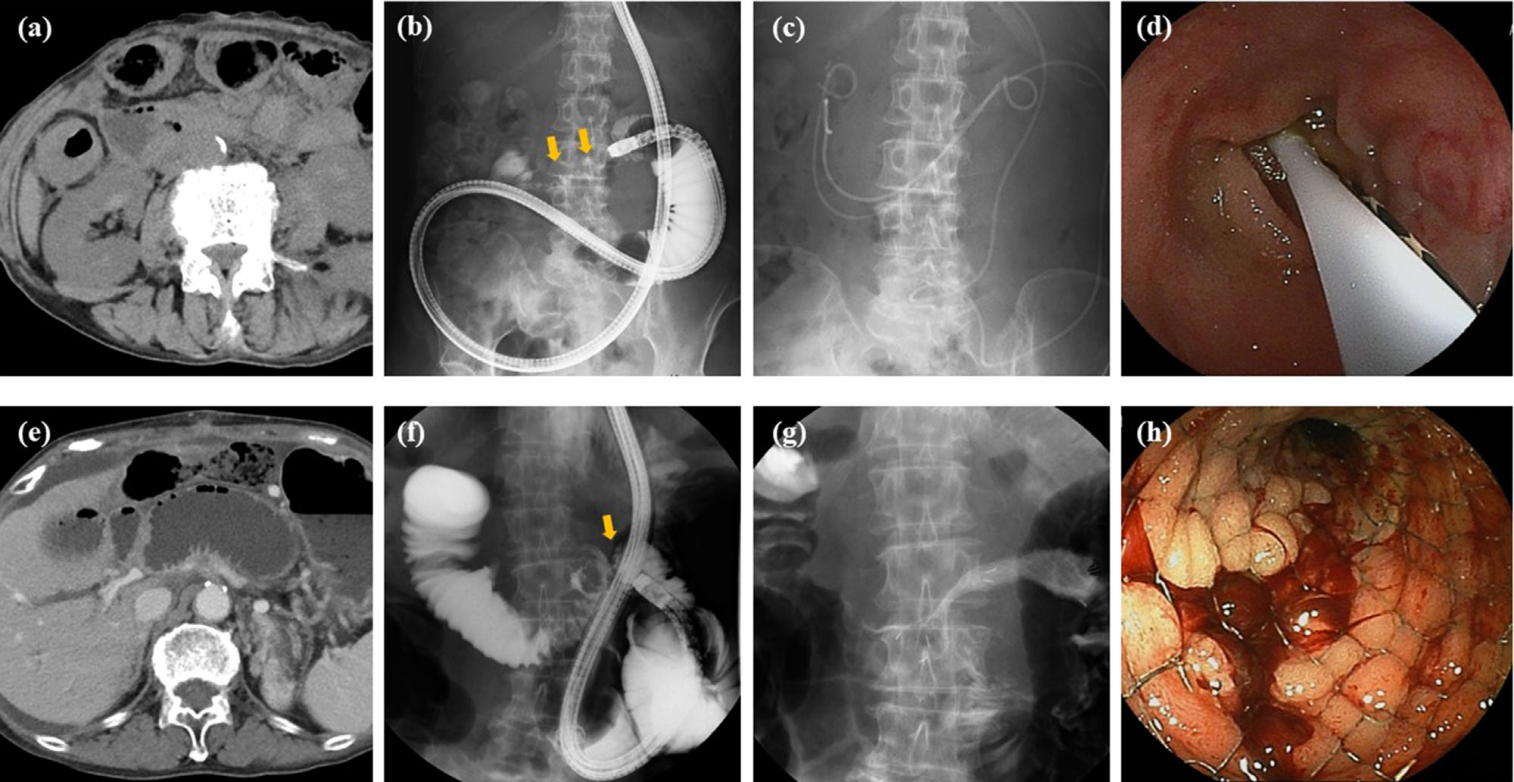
Double‐balloon endoscopic procedure for benign and malignant afferent loop obstruction (ALO). (a) Computed tomography (CT). CT imaging in benign ALO. (b, c) Fluoroscopy, endoscopic naos‐drainage, and endoscopic drainage using plastic stent for benign ALO. Intestinal stenosis (yellow arrow). (d) Endoscopy: endoscopic drainage using plastic stent for benign ALO. (e) CT: CT imaging in malignant ALO. (f, g) Fluoroscopy: endoscopic self‐expandable metal stent (SEMS) placement for malignant ALO. Intestinal stenosis (yellow arrow). (g) Endoscopy: endoscopic SEMS placement for malignant ALO
